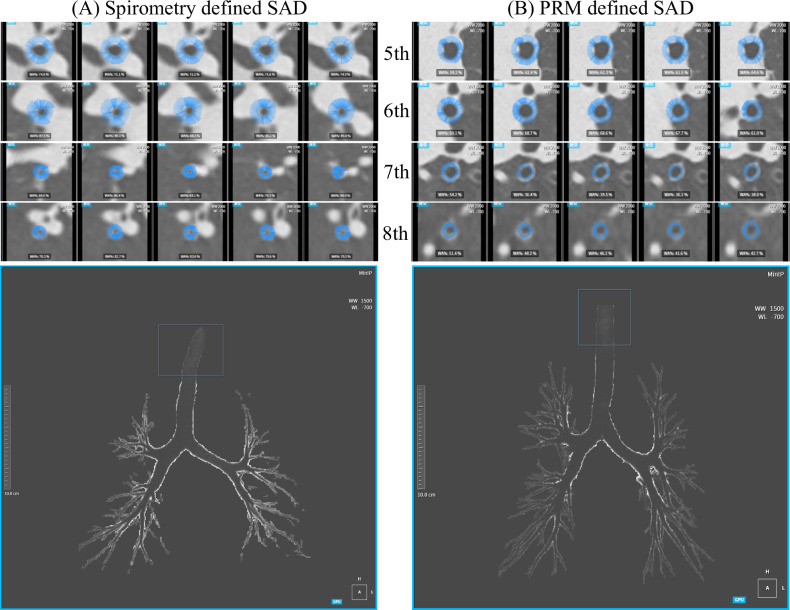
The upper part shows the cross-sections of the 5th to 8th generation bronchi as displayed by HRCT, with the blue rings representing the bronchial walls. The lower part shows the bronchial tree visualized by using the MinIP algorithm. A Shows a 67-year-old female participant with spirometry-SAD, with the average WA% of the 5th to 8th generations being 79.2%, 79.6%, 74.7%, and 69.1% respectively; B shows a 57-year-old female participant with PRM-SAD, with the average WA% of the 5th to 8th generations being 43.5%, 40.2%, 46.7%, and 43.1% respectively. SAD, small airway dysfunction; PRM, parametric response mapping; MinIP, minimum intensity projection; WA%, wall area percentage
